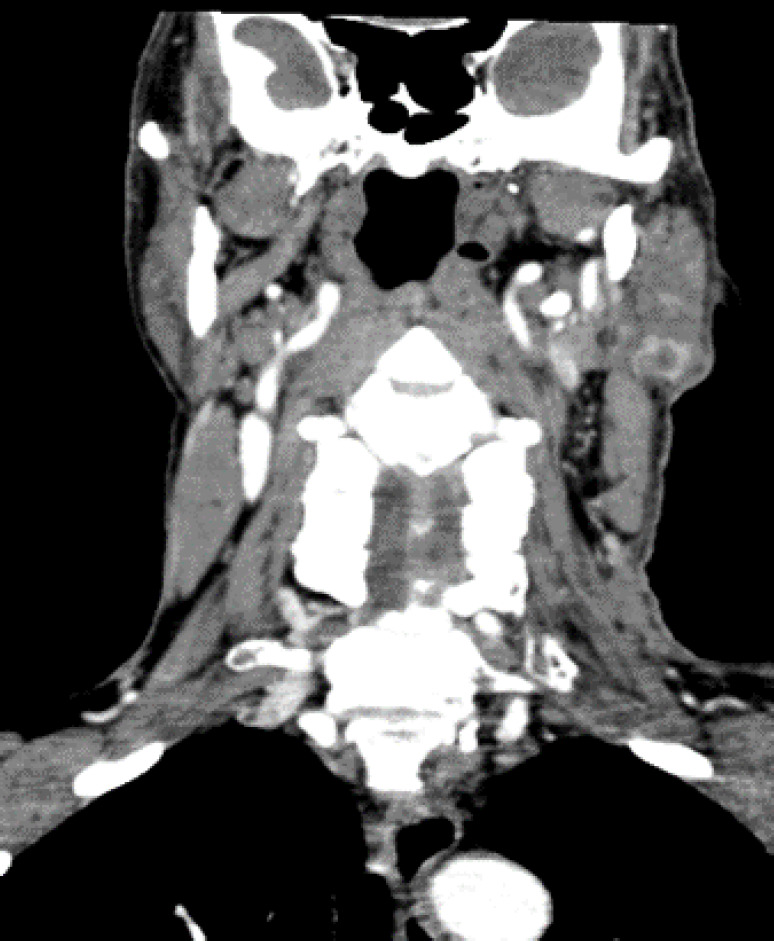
CT scan showed a heterogeneous enhancing mass measuring 20x12 mm in tail of the left parotid gland
